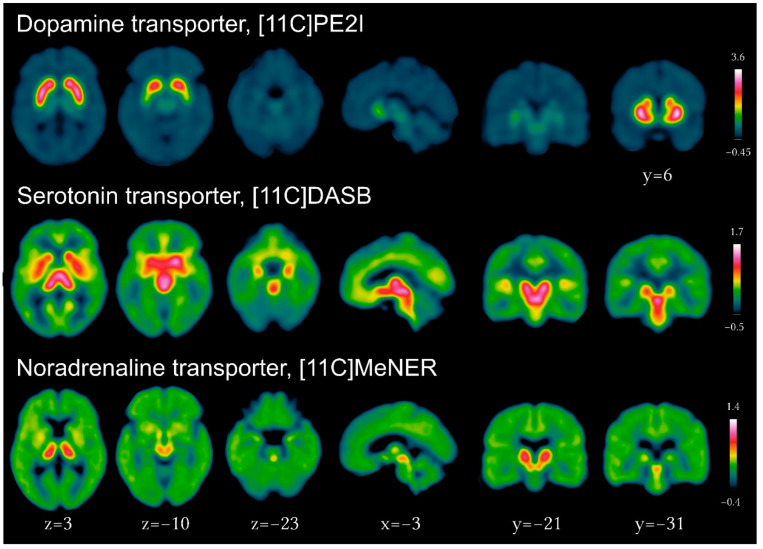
Imaging of the presynaptic dopaminergic, serotonergic and noradrenergic systems using PET tracers for the dopamine transporter ([11C]PE2I), serotonin transporter ([11C]DASB) and noradrenergic transporter ([11C]MeNER, courtesy of Christopher Doppler).
